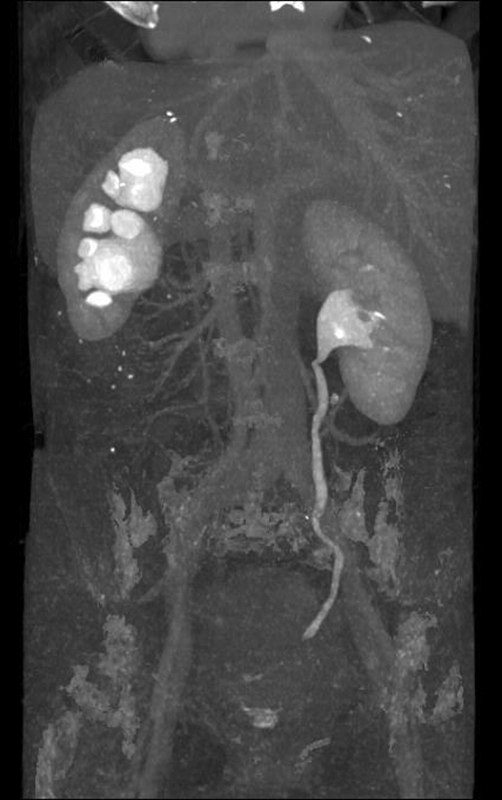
CT, September 2014. CT, computed tomography.
